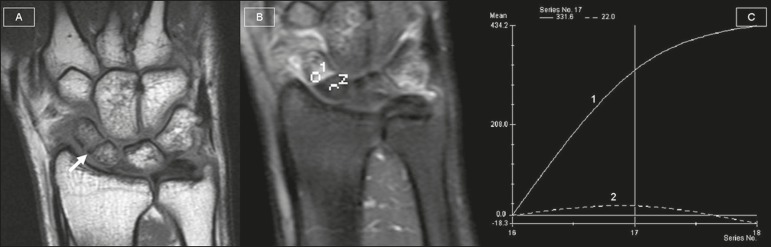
Coronal T1-weighted image showing a fracture line through the scaphoid waist
